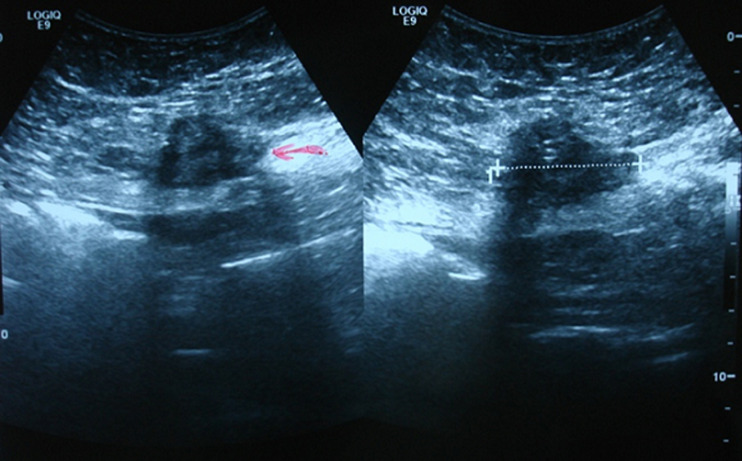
ultrasound sonography (arrow), 42mm round mass in the anterior abdominal wall
